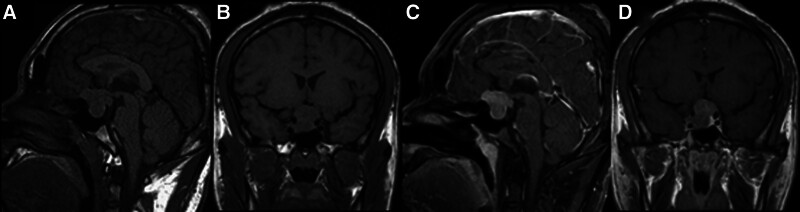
Patient MRI on November 18, 2019 (A and B: T1WI; C and D: enhanced MRI).
